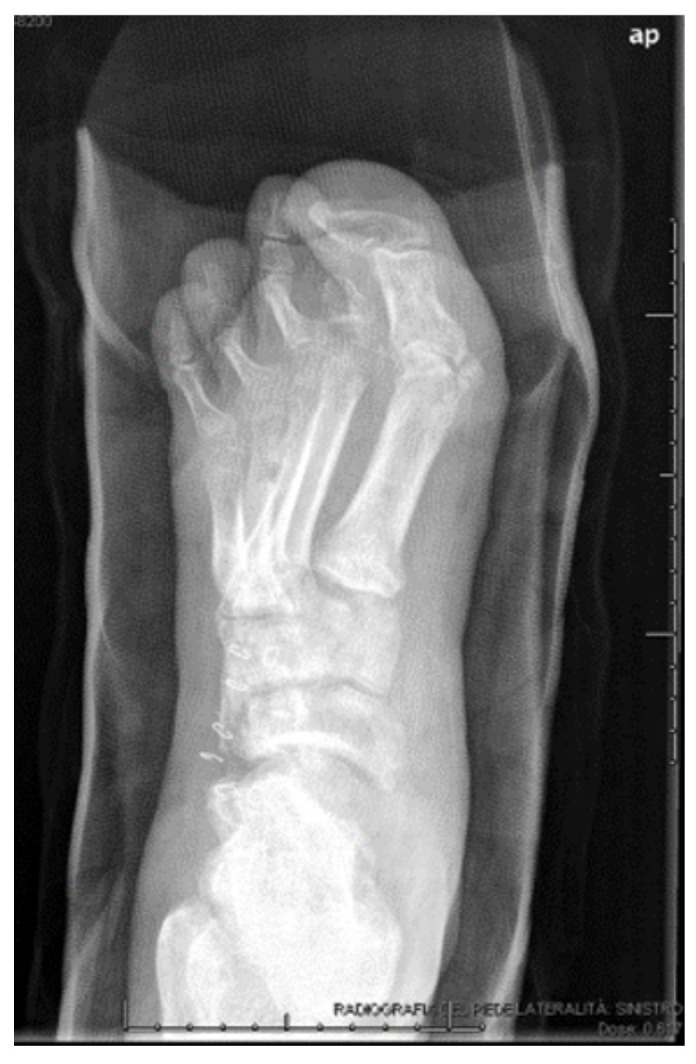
Radiographic outcome at 5.5 months.
